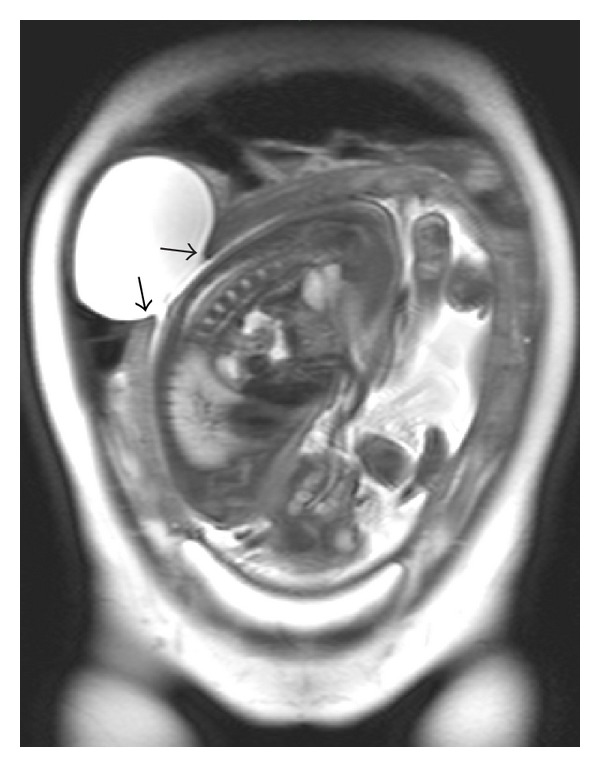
Magnetic resonance imaging (T2WI, coronal section) of the pelvis revealed a bulging amniotic cavity protruding through the defect in the uterine wall (arrows). Neither peritoneal fluid nor hemoperitoneum was observed.
